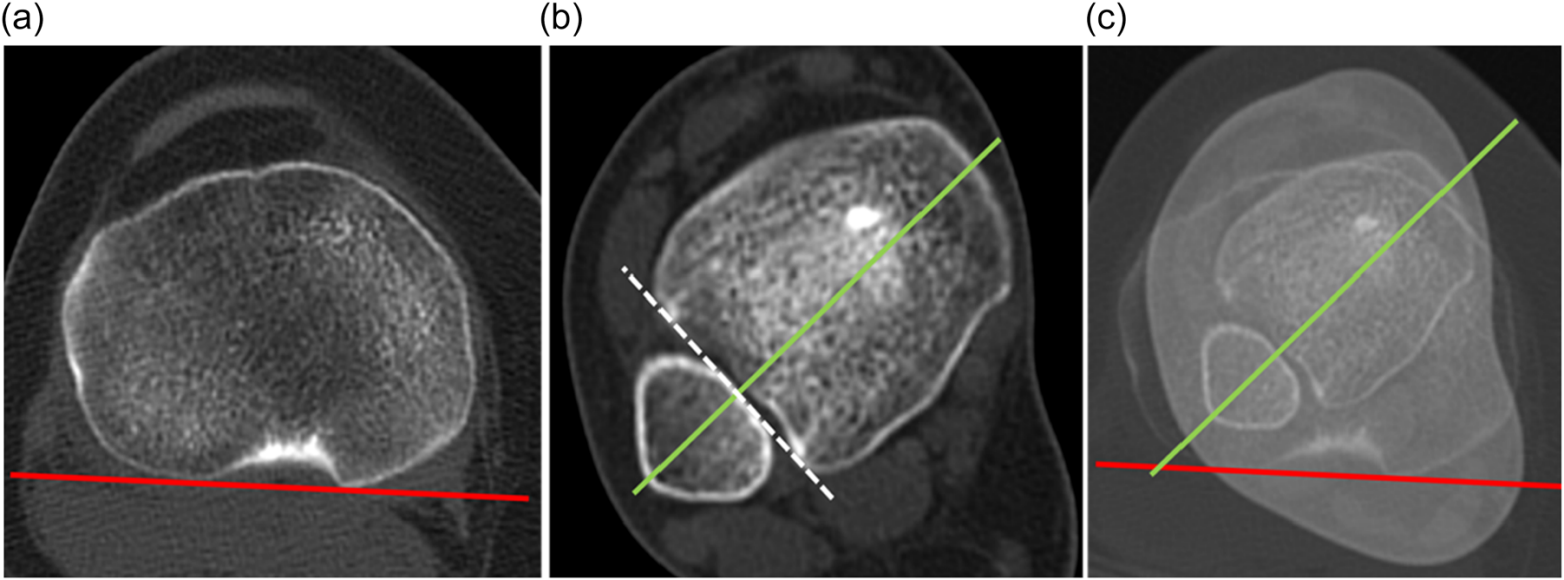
TTT measurement. (a) Axial CT image of the tibial plateau. The tangent to the posterior border of the tibial plateau forms the proximal line of reference (red line). (b) Axial CT image of the distal plafond and ankle malleoli. The line joining the most protruding part of the lateral and medial malleolus (green line), perpendicular to the fibular notch of the tibia (white dotted line) is the distal line of reference (green line). (c) Addition CT image of both tibial plateau and malleoli. Tibial torsion is the angle formed between the two lines. CT, computed tomography; TTT, total tibial torsion.
